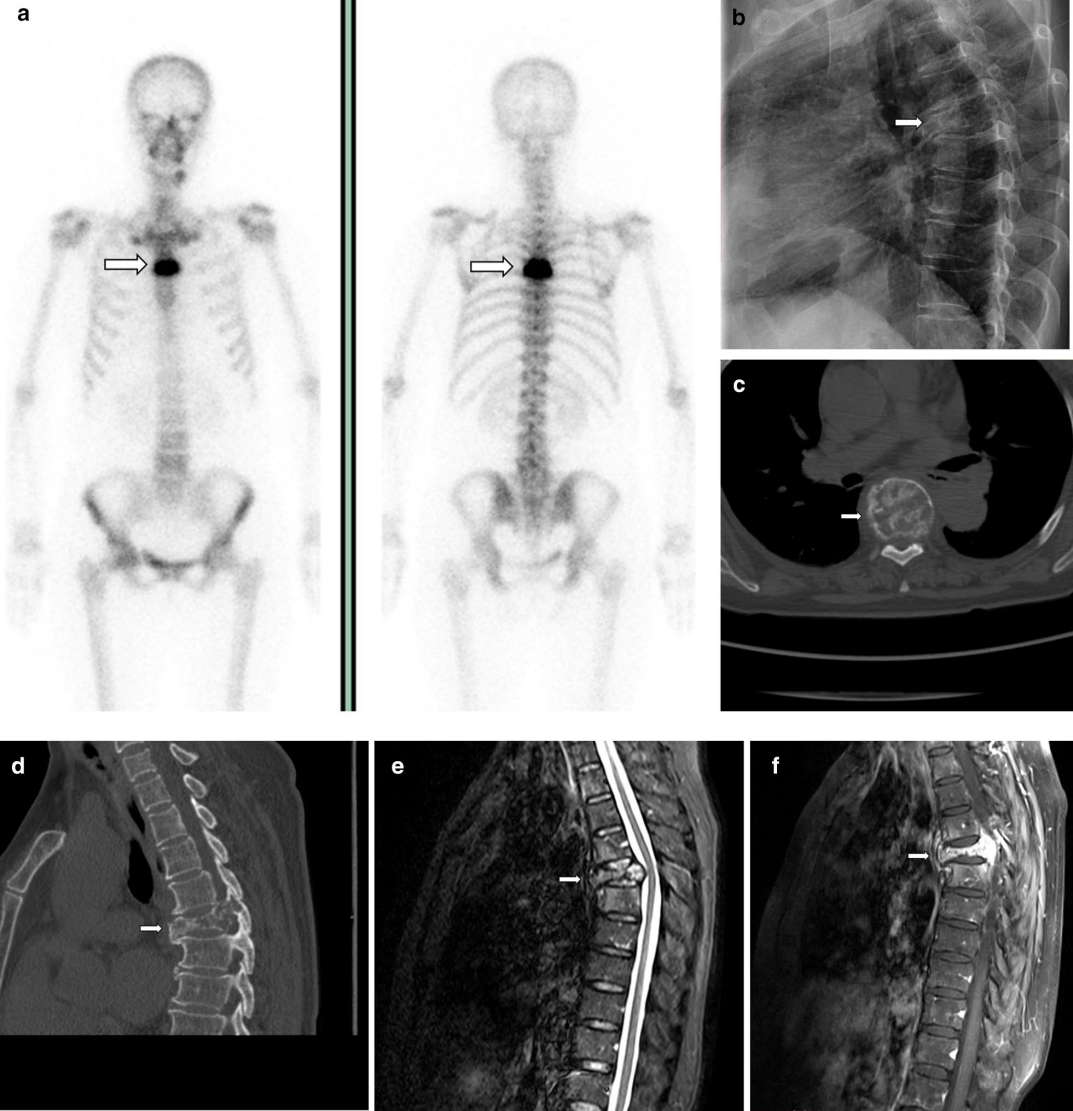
A 42-year-old man with osteoblastoma appearing as vertebra plana. Bone scan shows increased radionuclide tracer uptake at T7 (a). Radiograph shows a vertebra plana at T7 (b). CT depicts an expansile, mainly lytic soft tissue replacement of the bony trabeculae throughout the body (c, d). Pre- and paravertebral soft tissue extension, circumferential epidural extension enveloping and compressing the cord showed on T2WI (e) and contrast-enhanced T1WI (f)
